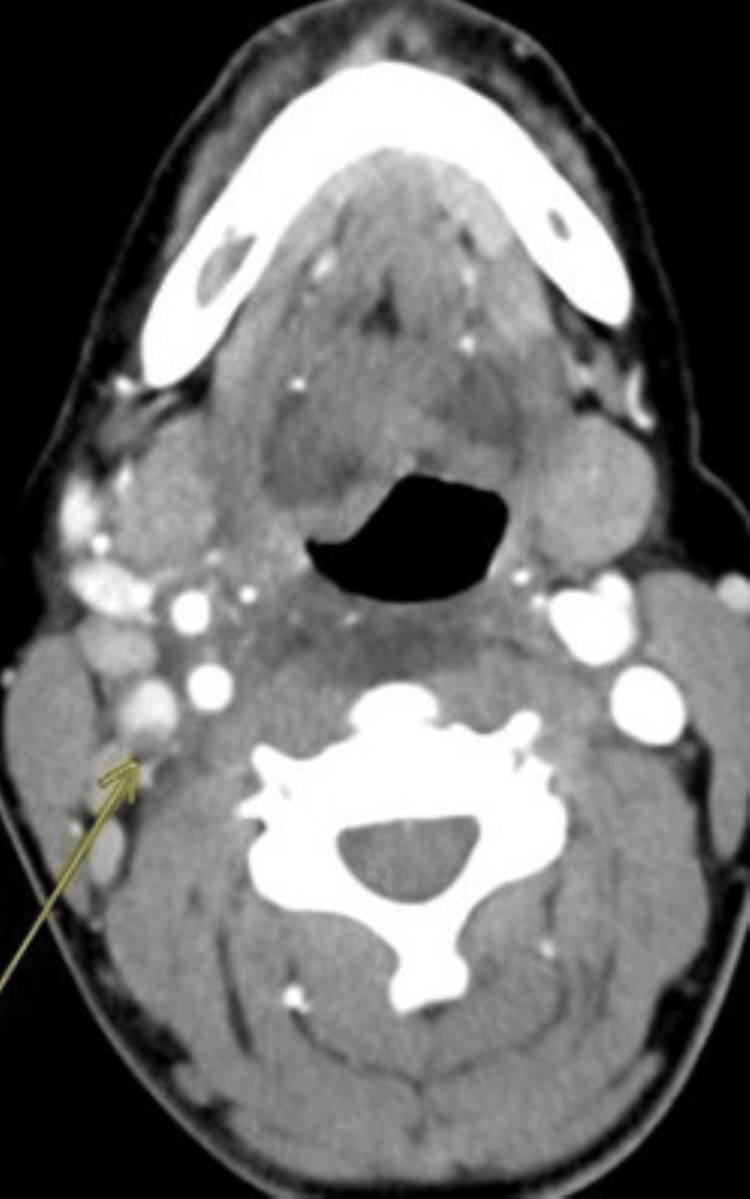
Axial view of CT with contrast demonstrating thrombus in the right IJV (yellow arrow). IJV, internal jugular vein
